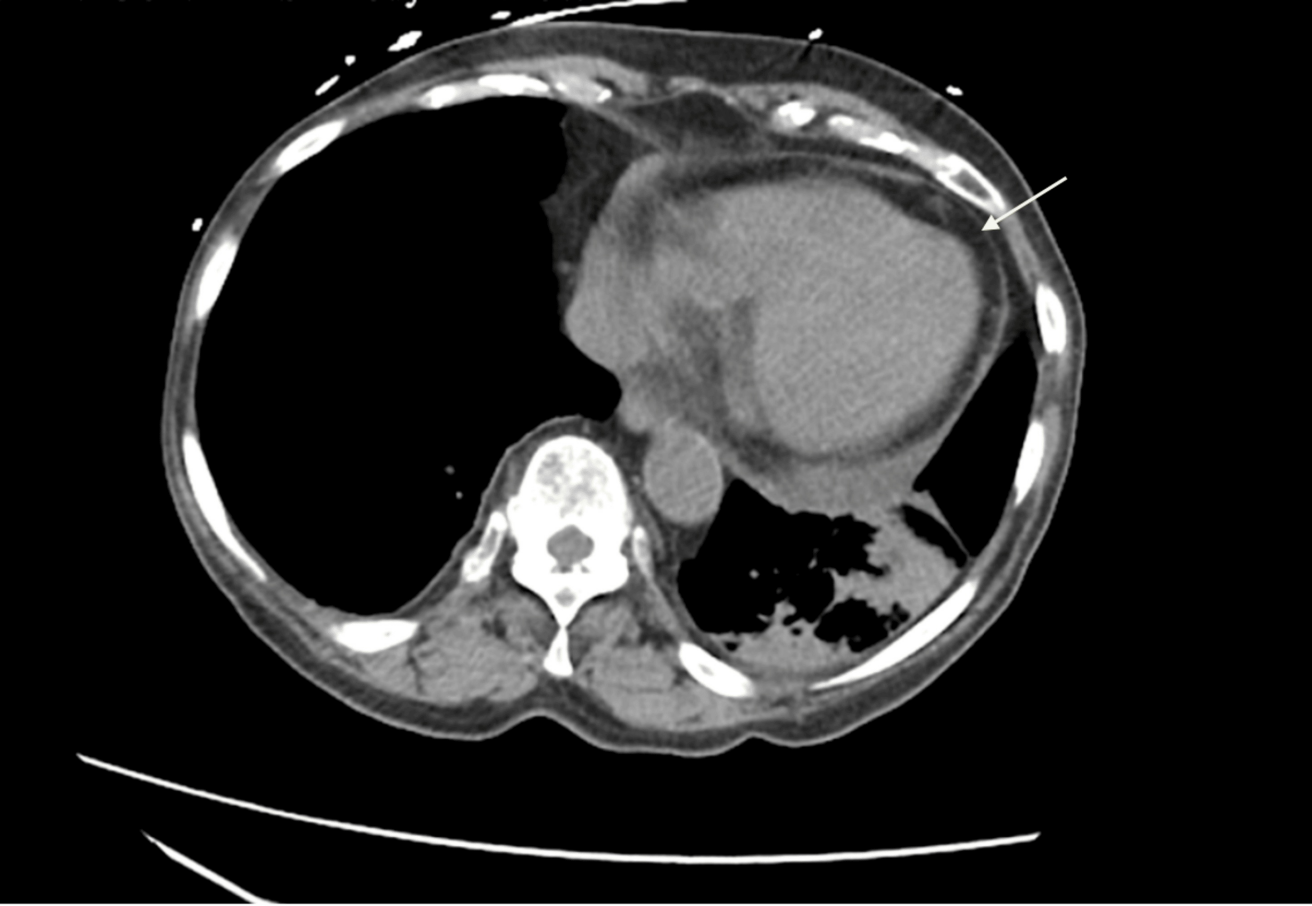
Axial view of the CT of the abdomen and pelvis without contrast shows pericardial effusion (maximum thickness of 1.5 cm along the left ventricle).
